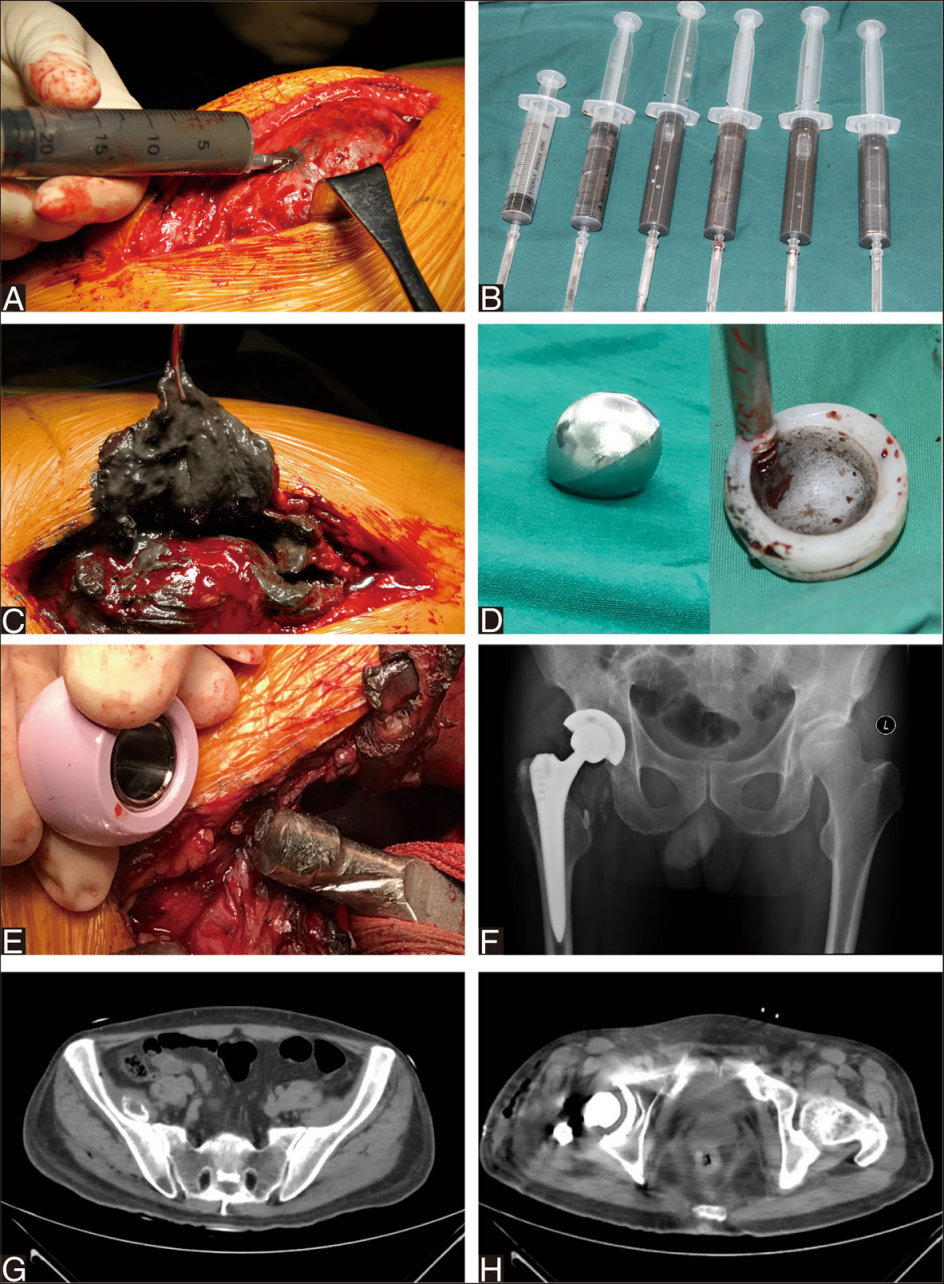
Re-revision CoP total hip arthroplasty for pseudotumor. a-b Dark gray drainage from surrounding synovial sacs was about 100 ml. c Dark gray tissue was visible around the right hip joint. d Photograph demonstrating metal head and polyethylene liner with severe wear. e A ceramic head along with a titanium sleeve was placed on the previous femoral stem. f-h Anteroposterior radiograph and transaxial view of the CT at immediate postoperatively showed unremarkable pseudotumor surrounding hip and a cystic wall in pelvis
